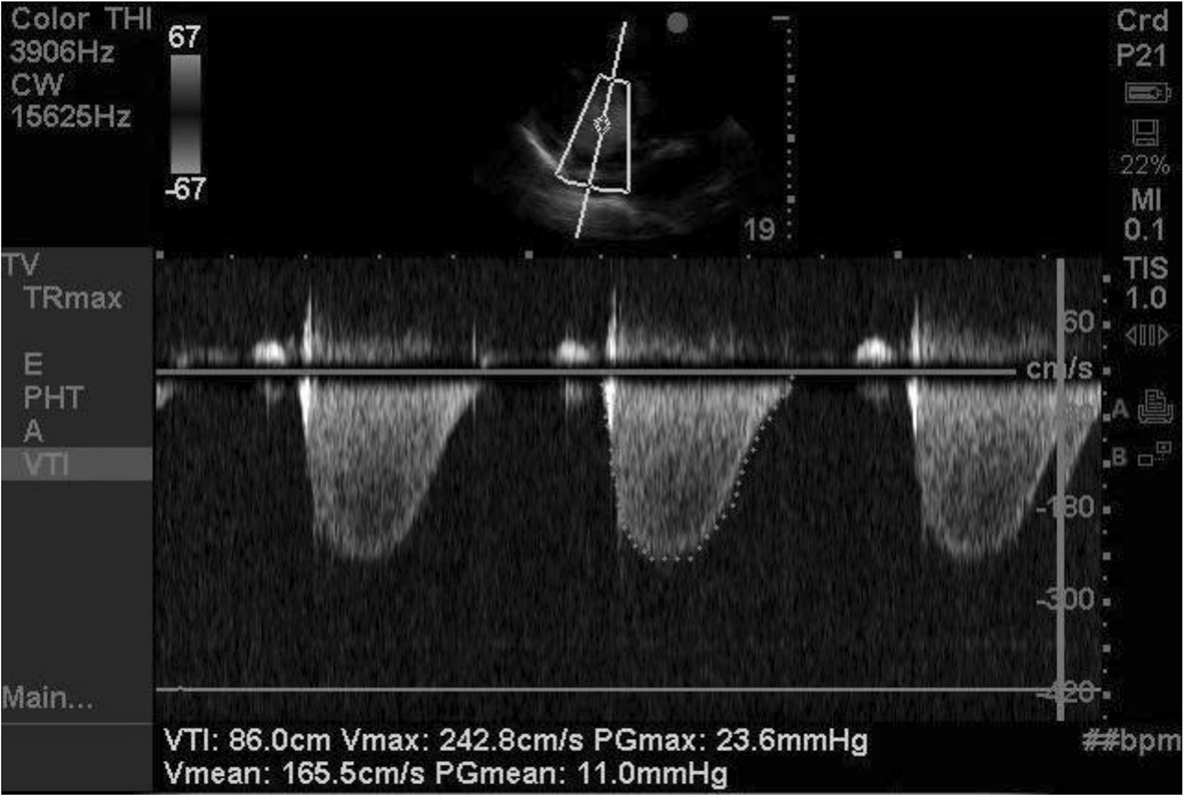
Echocardiographic estimation of pulmonary arterial pressures. Estimation of peak and mean right ventricular to right atrial systolic pressure gradients utilising continuous wave Doppler of the tricuspid regurgitant jet. Peak and mean pressure gradients were added to invasively measured right atrial pressures to estimate systolic and mean pulmonary artery pressures, respectively.
